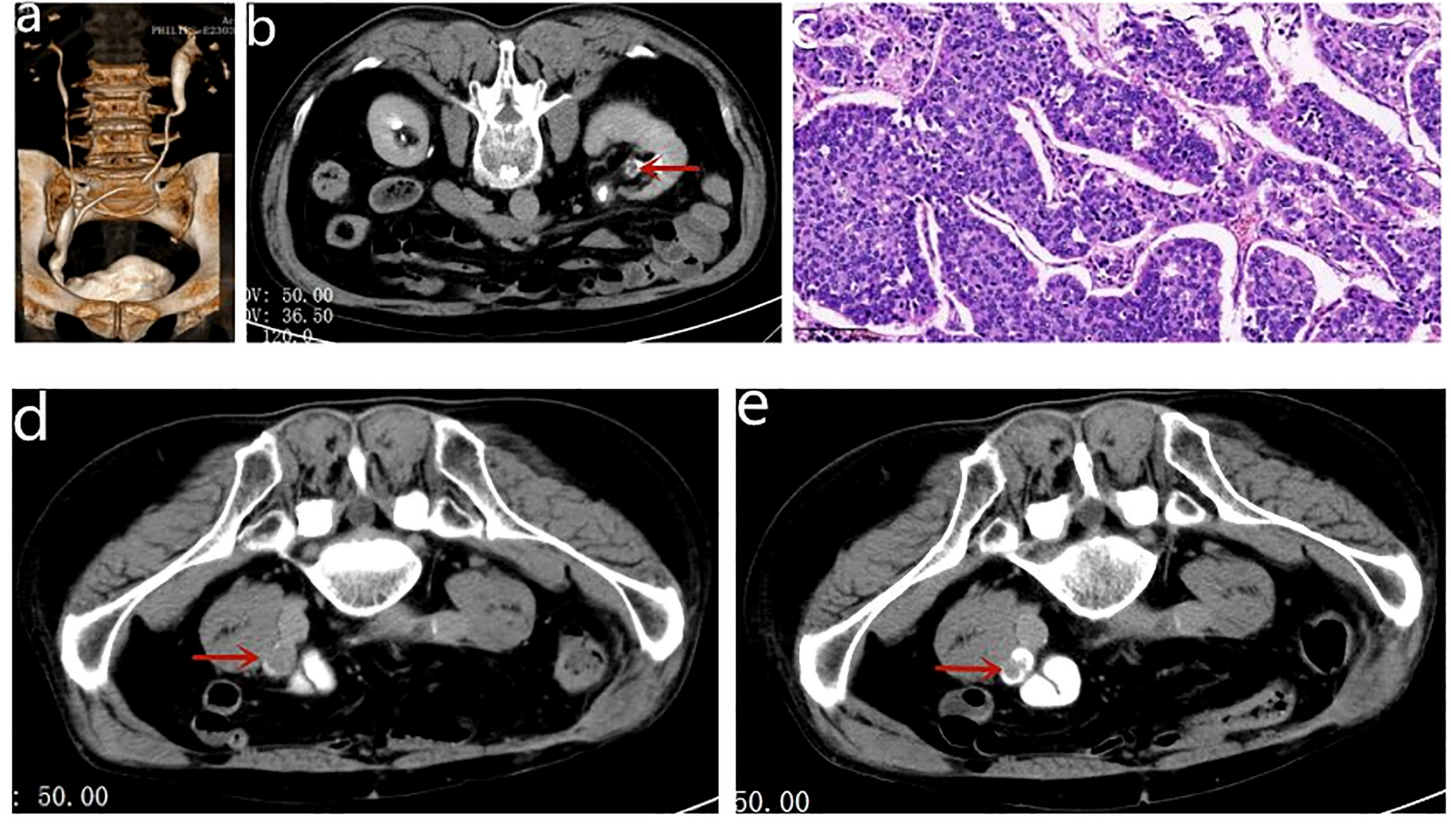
(A) CTU revealed that orthotopic neobladder was in good shape and there was no hydronephrosis in both renal pelvis. (B) The red arrows indicate the morphological changes in the left renal pelvis. (C) The pathological result identified high-grade urothelial papillary carcinoma of the left renal pelvis. (D) CT revealed recurrence of the right ureteral carcinoma with obstructive hydronephrosis. (E) CT revealed that the maximum diameter of the tumor shrank from 25 to 15 mm.
